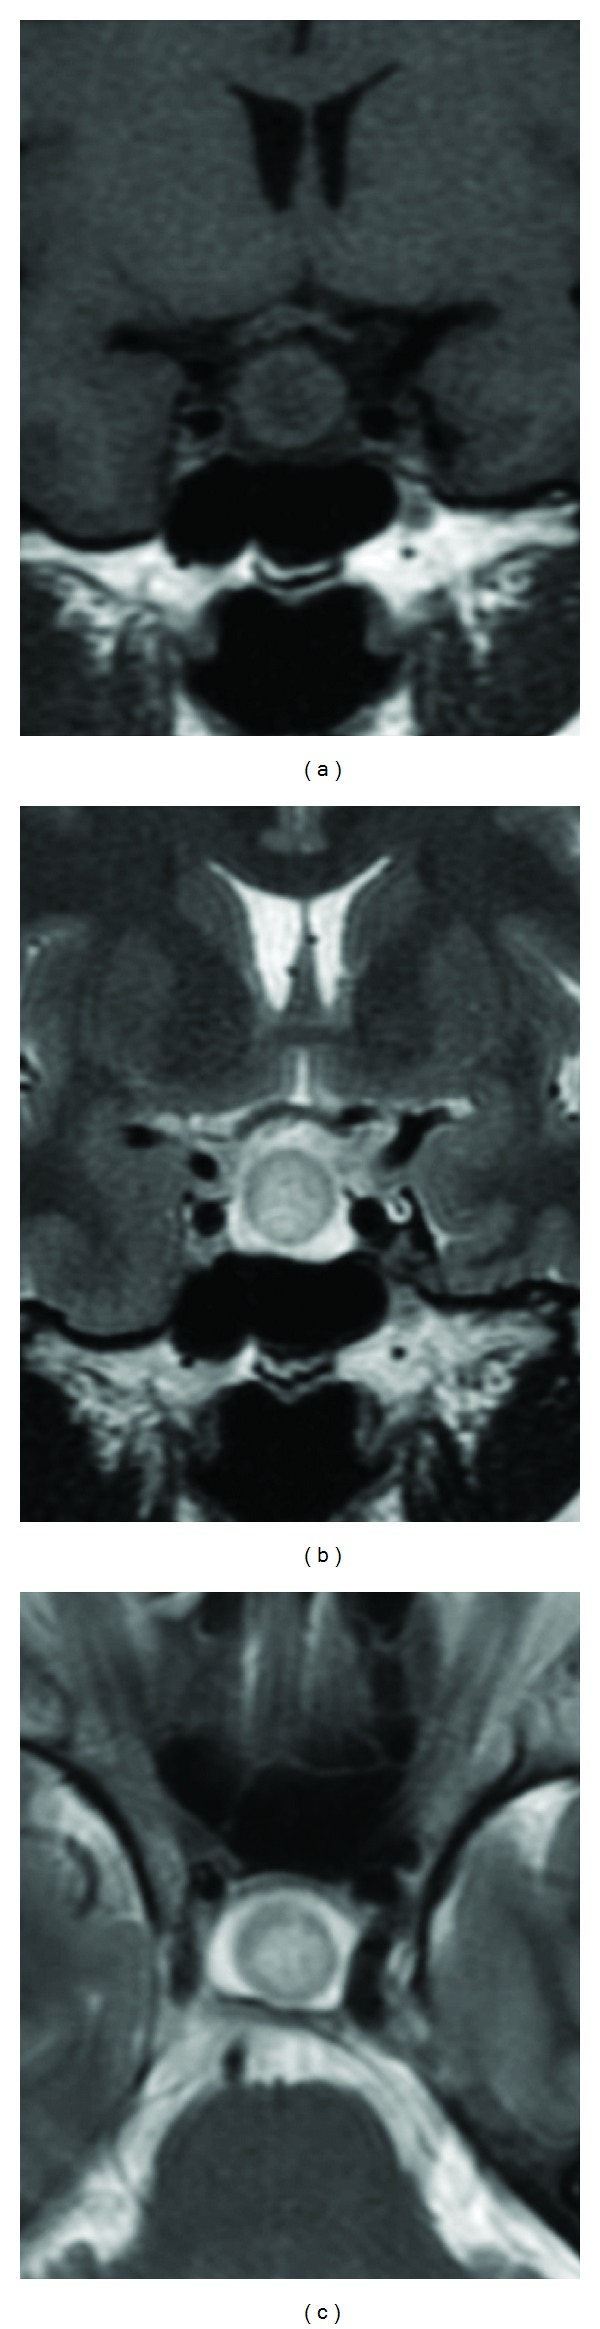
Case  10: nonenhanced MRI shows bull's eyelike intracystic nodules in RCC. Cyst fluid with hypointense signal in T1-weighted images and hyperintense signal in T2-weighted images. Circular isointense signal in T1- and T2-weighted images surrounding the nodule, and slight hypointense signal in T1-weighted image and slight hyperintense signal in T2-weighted images at the center of cyst. (a) nonenhanced coronal T1-weighted image; (b) coronal T2-weighted image; (c) axial T2-weighted image.
